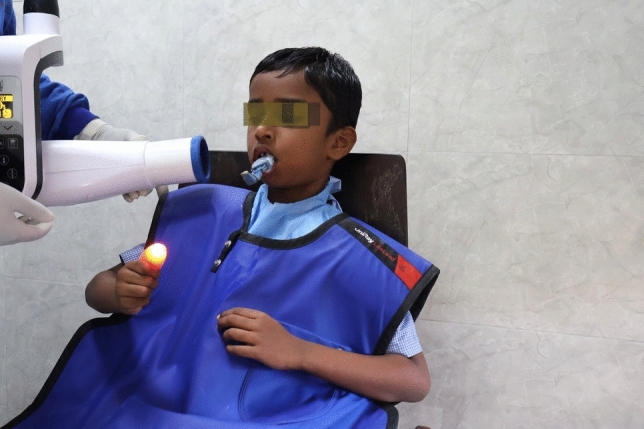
Taking an intraoral periapical radiograph while the child is playing with an acrylic thumb
